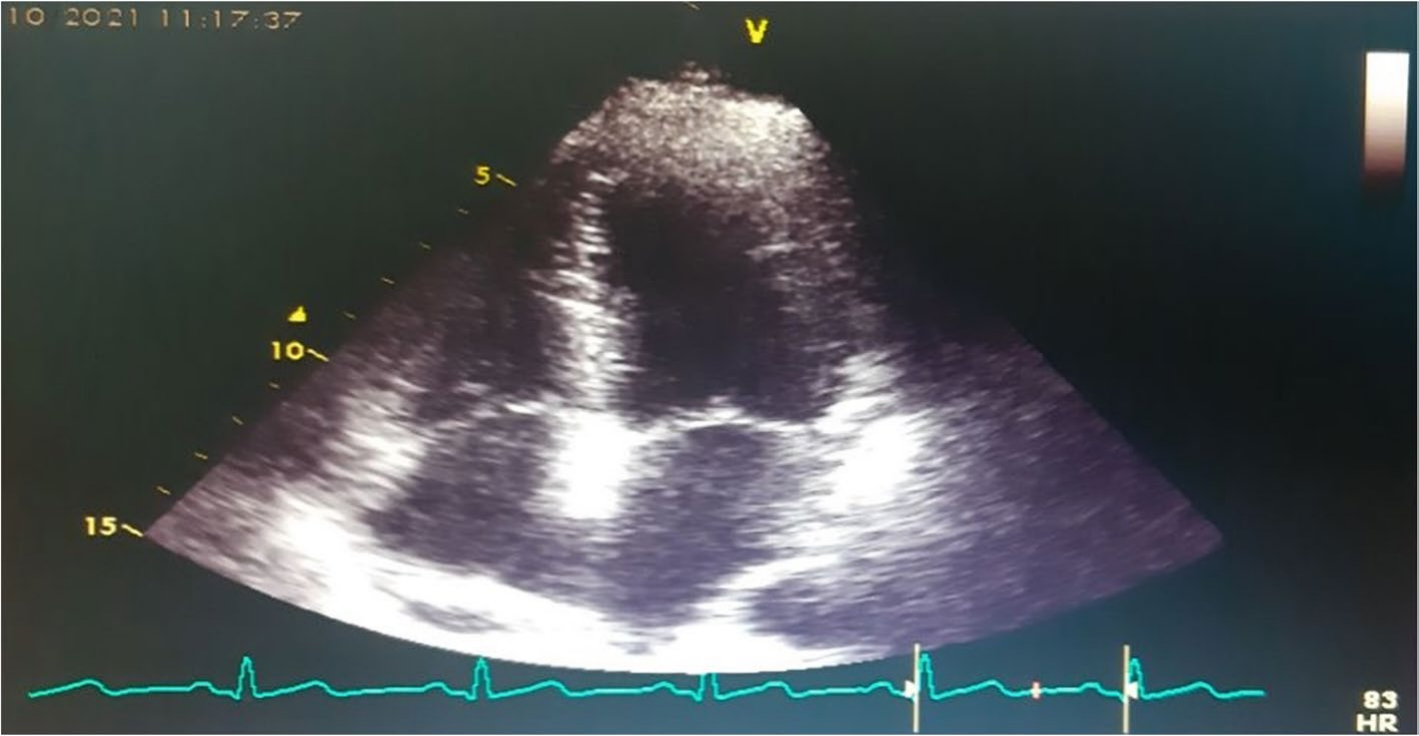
Epical view in transthoracic echocardiography after 72-h treatment showed complete resolution of thrombus
